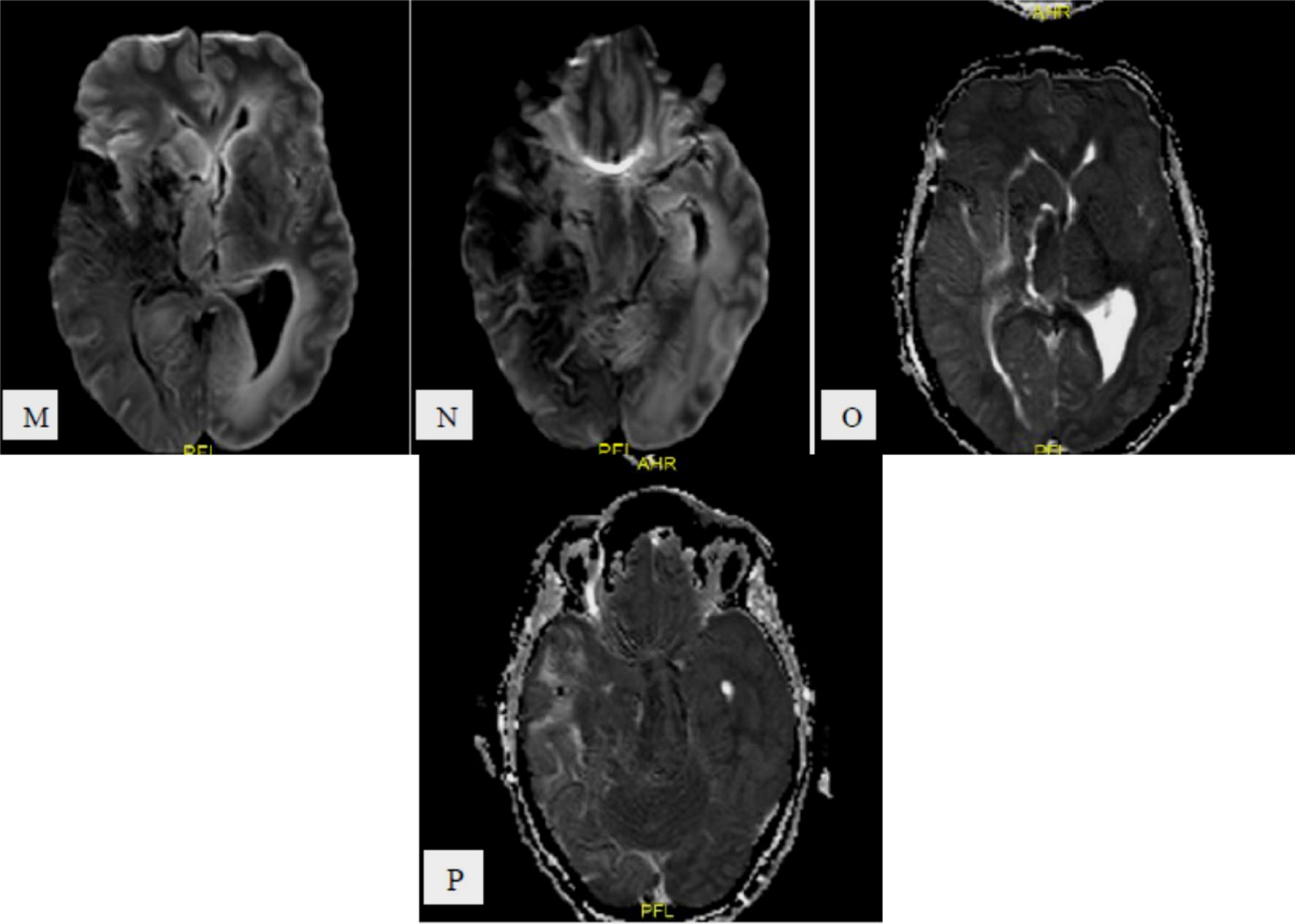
76-year-old COVID-19 male patient with sudden onset of left sided weakness (A) axial CT image shows right temporo-parital ill-defined deep white matter periventricular hypodensity, B, C Follow-up 2 days later of serial axial CT images shows progressive course regarding the size of the right fronto-temporo-parieto-occipital large right cerebral infarction with hemorrhagic component with sub-falcine herniation. D, E T1 WI, F, G T2 WI, H, I FLAIR, J–L SWI, M, N DWI, O, P ADC map, 7 days later MR follow up shows *aging*
